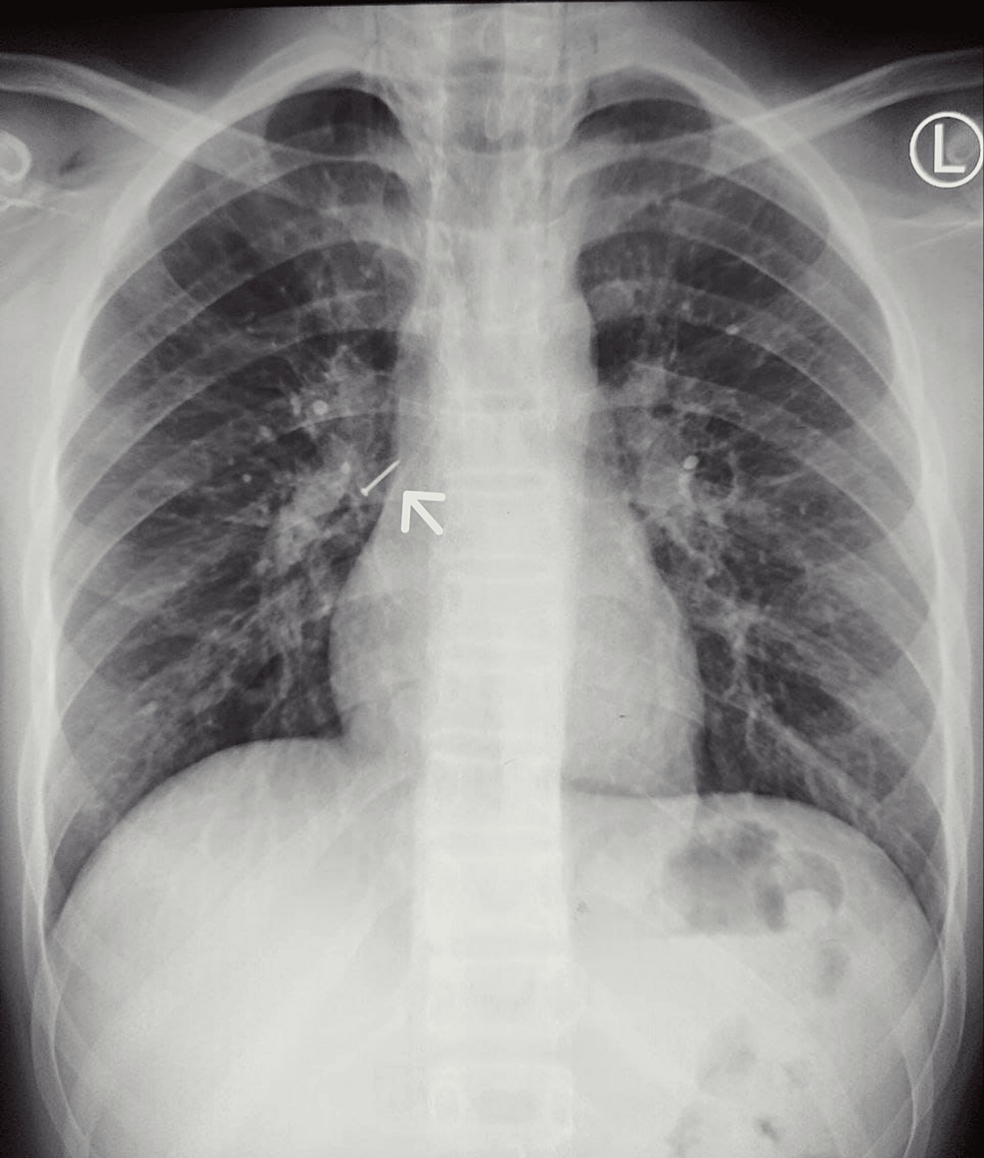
Chest radiograph showing a clear-cut radiopacity over the right main bronchus, suggestive of a pin (white arrow)
